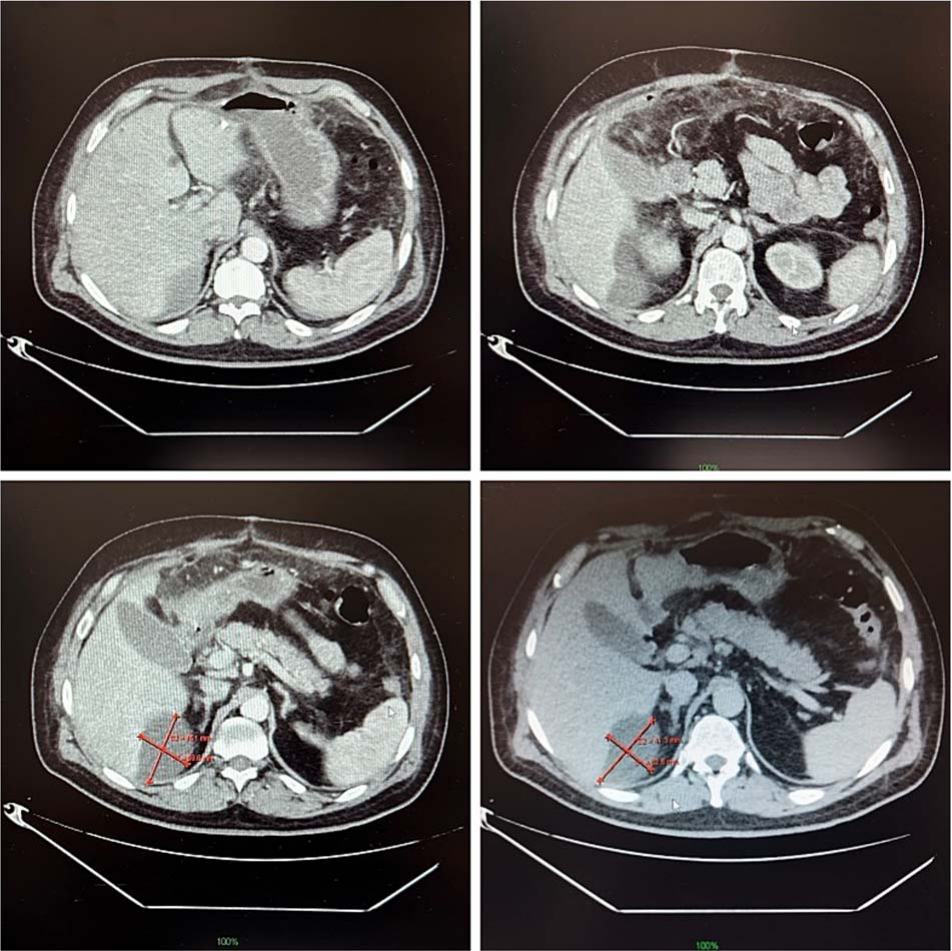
Contrast-enhanced abdominal CT on postoperative day 14, demonstrating a 9 × 5 cm abscess in segment VII.
